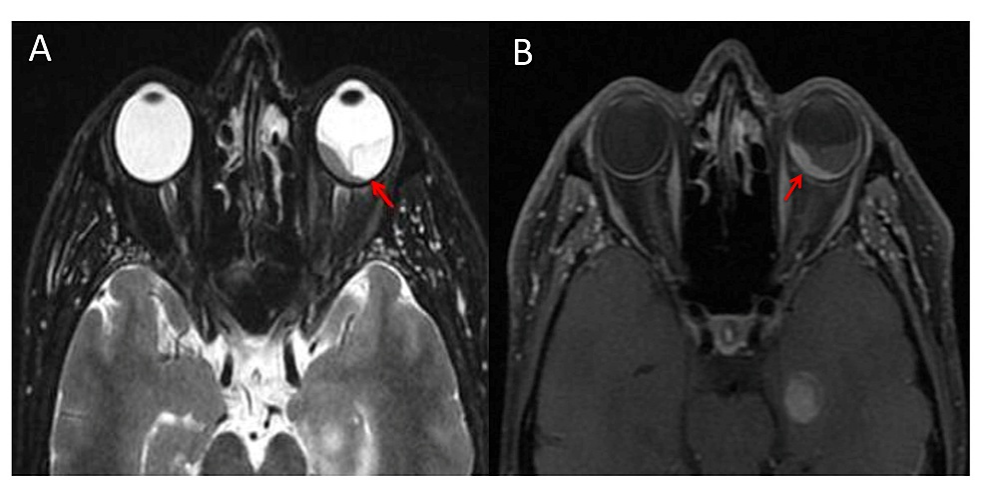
Axial T2 (A) and T1 weighted fat saturation after Gadolinium administration. (B) Magnetic resonance images showing choroidal lesion and retinal detachment of the left eye.
